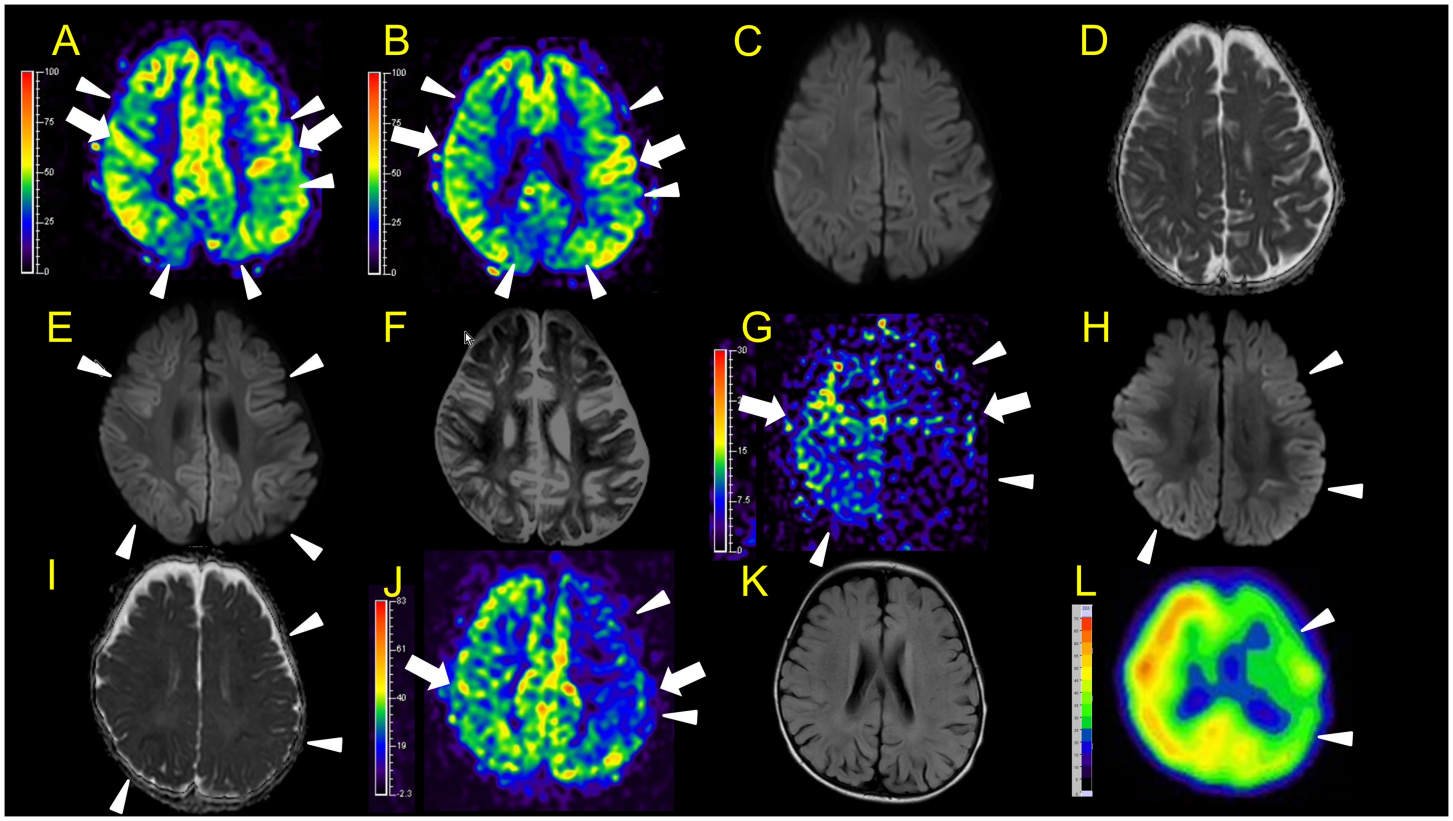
Neuroimaging findings. Color bars for ASL and SPECT indicate signal intensities, not absolute perfusion values. Patient 3 (A–F): A, B, ASL (A and B, post-labelling delay 1,500 ms, B is a lower slice of A), DWI (C), ADC (D) at 46 h after ESs. ASL (A: arrowheads) revealed hypoperfusion areas compared to perirolandic regions (arrows) in the bilateral frontal, left parietal, and bilateral occipital regions without DWI or ADC abnormalities (C,D). E,F, DWI, and ADC on day 12. Hypoperfusion areas in bilateral frontal and parietal lobes in ASL at 46 h after ESs (A: arrowheads) coincide with BTA (E: arrowheads) on day 12. Patient 4 (G–L): G–I, ASL (post-labelling delay 1,500 ms), DWI, ADC at 104 h after ESs. ASL image obtained in the subacute phase revealed hypoperfusion in the right frontal and parietal regions, and the entire left hemisphere (G: arrowheads) compared to the right perirolandic region (arrows). Broad hypoperfusion areas in the left hemisphere were seen. DWI and ADC revealed slight BTA in the left frontal and bilateral parietal regions (H,I: arrowheads). (J,K) ASL (post-labelling delay 1,500 ms) and FLAIR on day 30. L, SPECT (with 99mTc-ECD) on day 43. ASL images and SPECT acquired in the subacute phase persistently showed hypoperfusion areas in the left hemisphere (J,L: arrowheads) compared to the right perirolandic region (J: arrow) without abnormal FLAIR findings.
